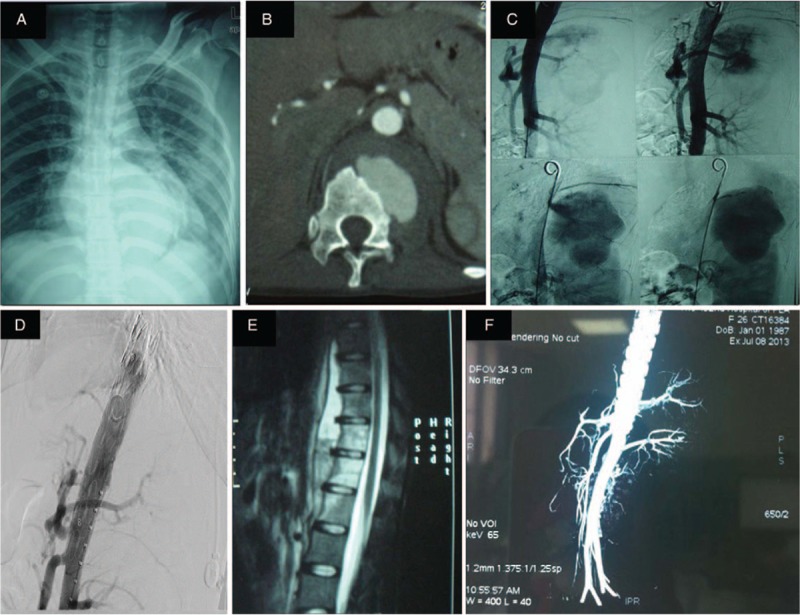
Patient 3: Plain radiography (A) showed T6–T10 bone destruction with paraspinal abscess formation. Enhanced computed tomography (CT) (B) and angiography (C) show the pseudoaneurysm on descending aorta. Endovascular stent grafting (D) was performed to treat the aneurysm, and repeated angiography confirmed a complete closure of the mass. The patient had good stability of the spine (E). After 18 months's follow-up, repeated CT (F) showed no pseudoaneurysm recurrence.
